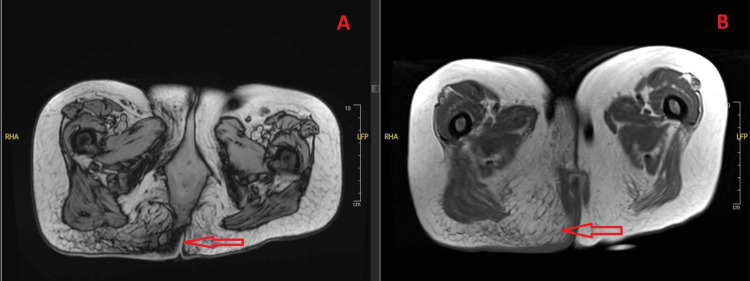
Axial pelvic MRI (A) Axial T2-weighted MRI demonstrates a presacral/perianal fluid collection with peripheral rim hyperintensity (arrow), consistent with an abscess, extending into the right gluteal region. Associated surrounding fat stranding and soft tissue edema are also evident; (B) A more caudal axial slice shows further extension of the fluid collection into the perianal soft tissues (arrow), with asymmetric involvement of the right buttock, correlating with the site of the patient’s ulcerated plaque.
